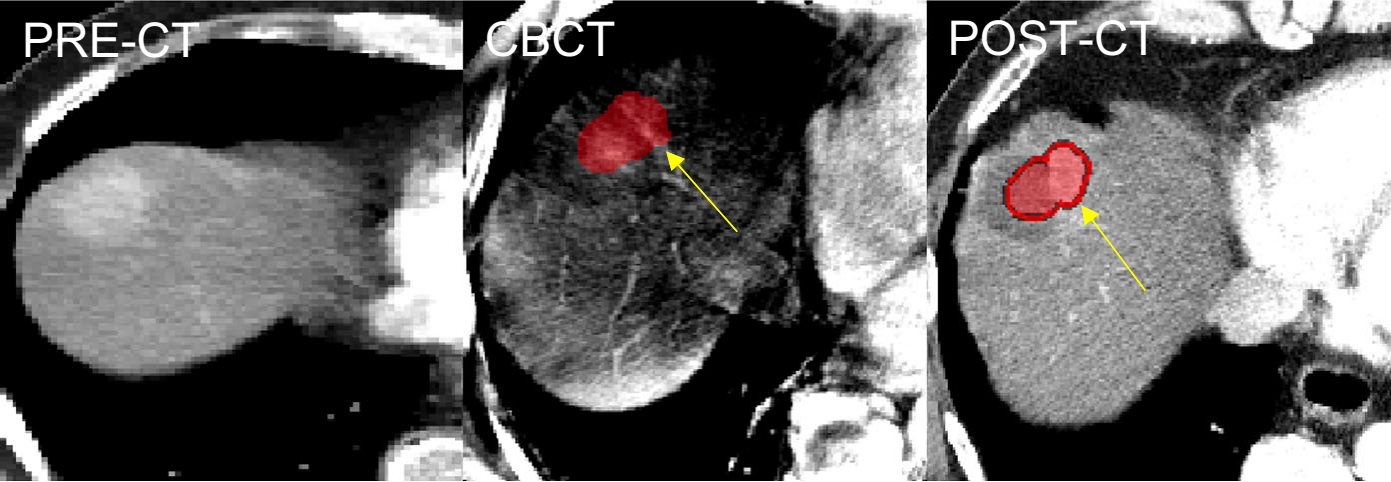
Incomplete treatment detected by CBCT. Intraprocedural CBCT registered with PRE-CT in patient #8 of Table 1 shows partial failure of the ablation treatment, as confirmed by the 24 h post-CT. The medial portion (yellow arrows) of the HCC (colored in red) is not entirely covered by the ablated area both on CBCT and on post-CT
